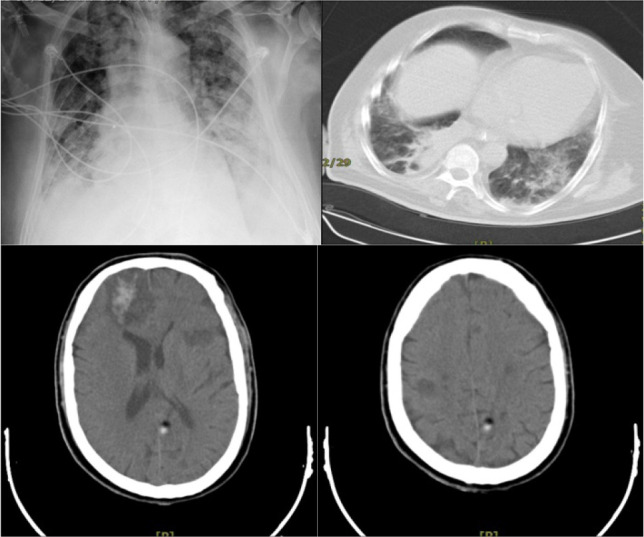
Pattern of pulmonary and brain involvement of patient CT scan
